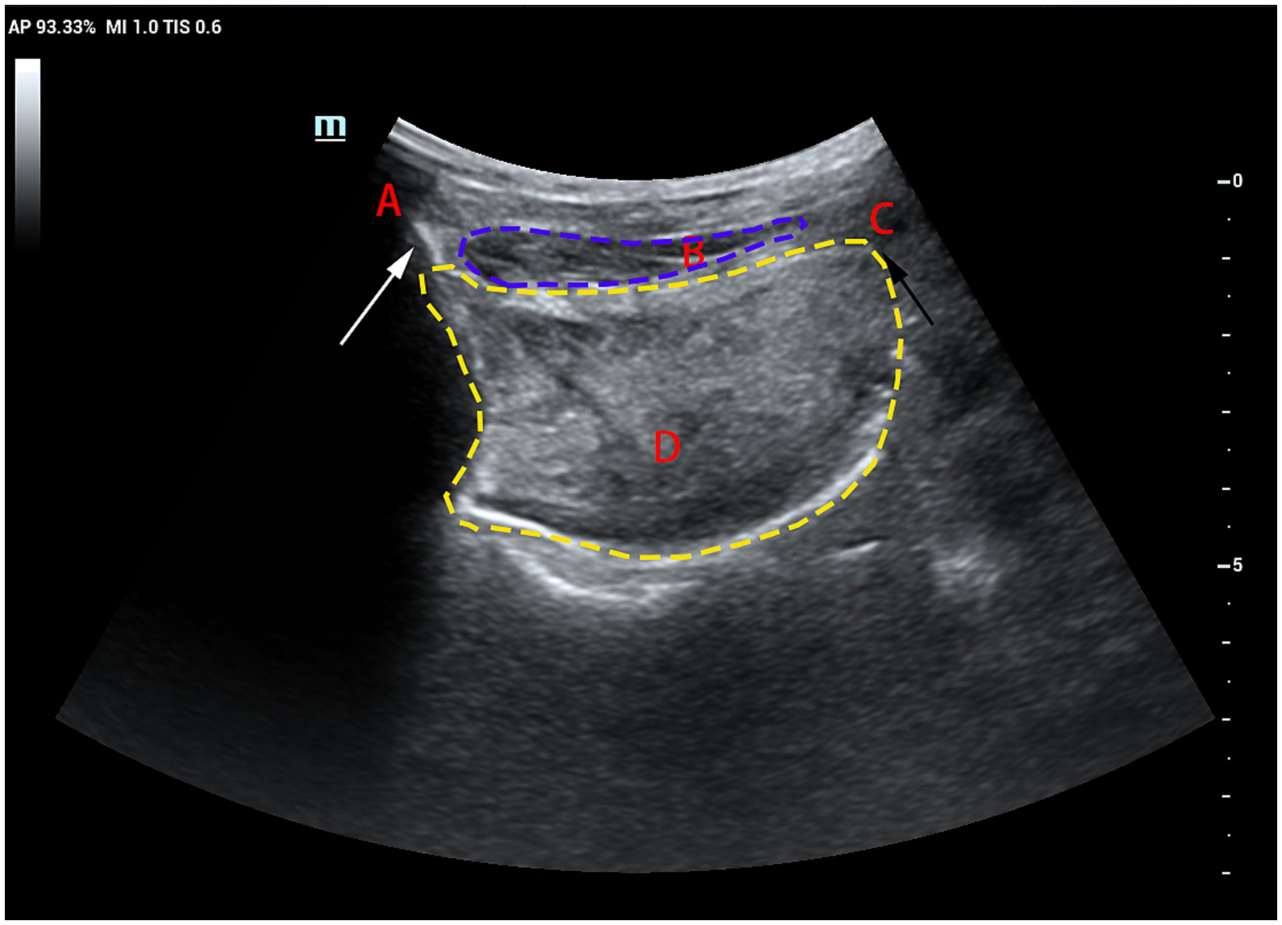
B-mode ultrasound image showing the genioglossus and the hyoid bone in the median sagittal position (A) mandible: as indicated by the white arrow; (B) genioglossus: as indicated by the blue dotted curve; (C) hyoid: as indicated by the black arrow; (D) tongue: as indicated by the yellow dotted curve.
